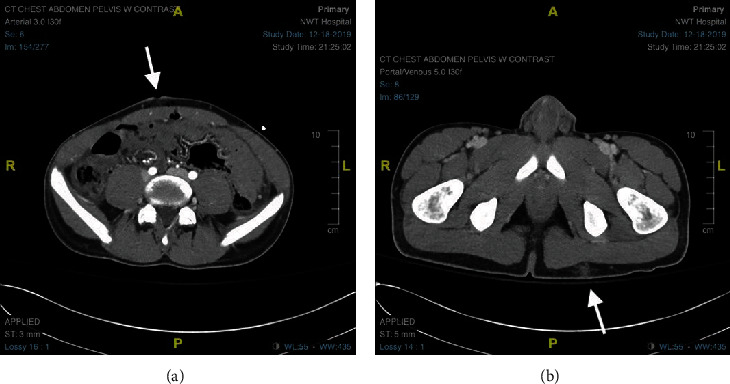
(a) CT scan of the abdomen/pelvis with bullet entrance point. (b) CT scan of the abdomen/pelvis with bullet exit point.
